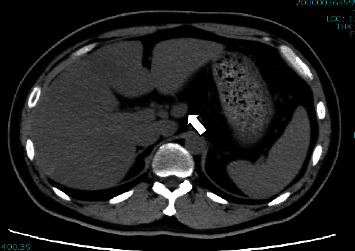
Plain abdominal CT on day 2 of hospitalization showed mild splenomegaly (arrowhead) and intra-abdominal lymphadenopathies (arrow).
